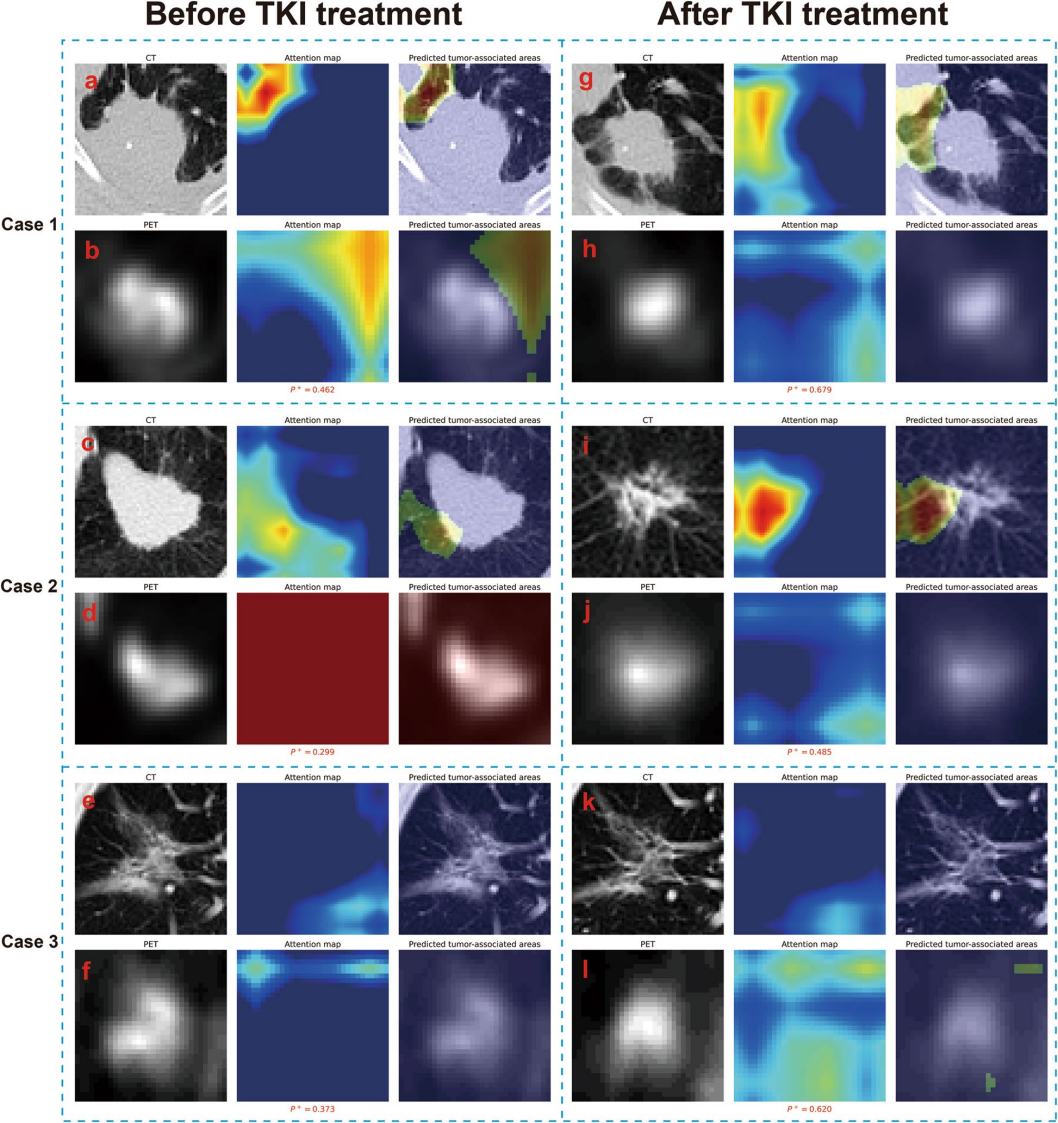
The mutant type-associated activation areas of the TS_TL in three EGFR-mutant lesions before and after TKI treatment. Case 1 (stage IV): A female non-smoker in the 60–65 age range presented with a solid mass in the left lower lung (41.1 × 29.7 mm) with an EGFR mutation in exon 20 and received oral poziotinib treatment; through PET/CT re-examination after 21 months, the original lesion shrunk (30.2 × 24.6 mm) and its metabolism was lower than before (SUVmax from 14.8 to 10.2). Poziotinib is a novel targeted drug for rare insertion mutations in exon 20 of EGFR and HER2. Case 2 (stage IV): A male smoker in the 65–70 age range presented with a solid mass in the left upper lung (40.9 × 26.7 mm) harboring an exon 21 EGFR mutation. The patient received oral Osimertinib treatment, and after 6 months, a follow-up PET/CT examination revealed a significant reduction in the size of the original lesion, which became subsolid (20.7 × 15.9 mm), and a decrease in metabolism (SUVmax from 11.8 to 3.9). Case 3 (stage IV): A male non-smoker in the 80–85 age range presented with a subsolid mass in the right upper lung (35.5 × 30.5 mm) carrying an exon 19 EGFR mutation. The patient received oral Icotinib hydrochloride treatment, and after 46 months, a follow-up PET/CT examination revealed a slight reduction in the size of the original lesion (28.5 × 27.9 mm) and a decrease in metabolism (SUVmax from 3.1 to 1.9)
